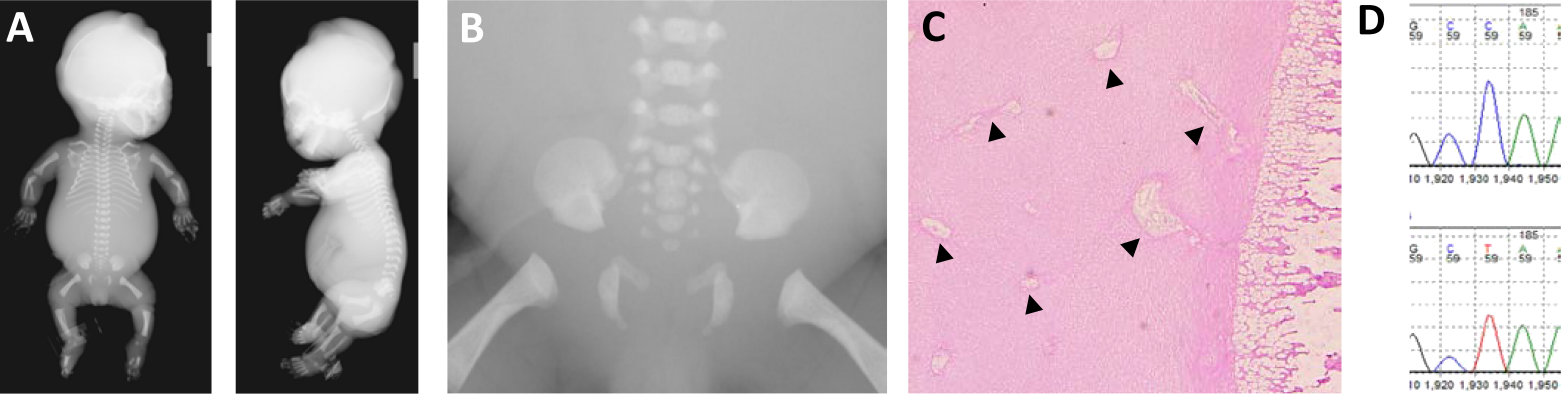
Phenotype and genotype of the affected fetus (MCA 4) (A) Radiographs of the proband (MCA 4). (B) Radiograph outlining the absence of a snail-like ilia in the proband (MCA 4). (C) Epiphyseal plate of femur, featuring an increase in vascular channels within the resting cartilage (indicated by arrows). (D) Sanger sequencing of the homozygous c.398C > T variant in the affected fetus.
